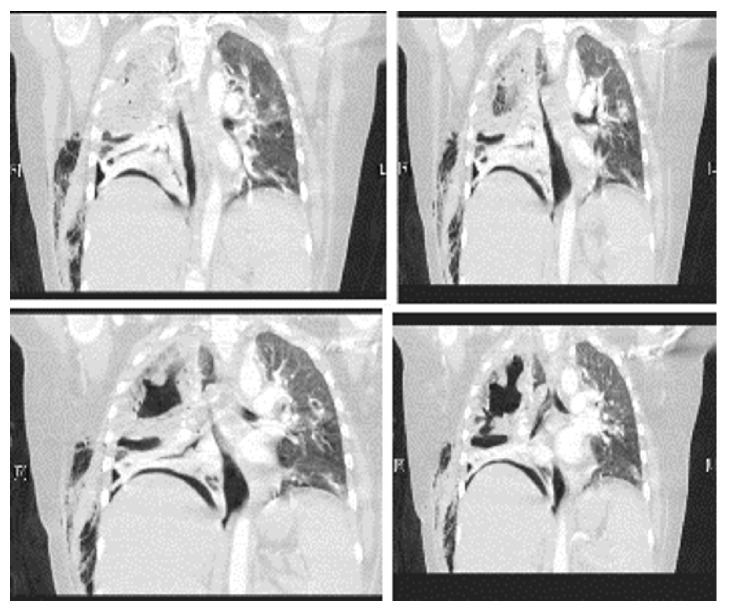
Chest computed tomography revealed multiple bilateral cavitation (more on the right), moderate right-sided pleural effusion, air-space infiltrates, and a right-sided cavitary-pleural communication.
